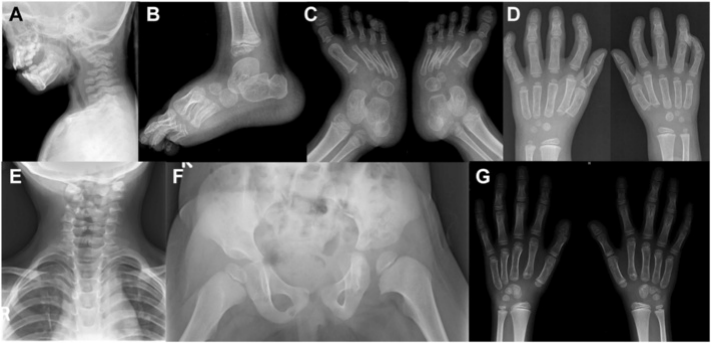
Radiographs of patient 3. She had cervical kyphosis with subluxation at C3-C4 (a), accessory calcaneal epiphysis (b), club feet with metatarsus adductus (c), advanced carpal ossification at 22 months (d), failure of fusion of posterior element of cervical spine with mild scoliosis (e), normal right hip joint with increased medial joint space on left hip joint (f), and normal bone at 4 years 7 months (g)
